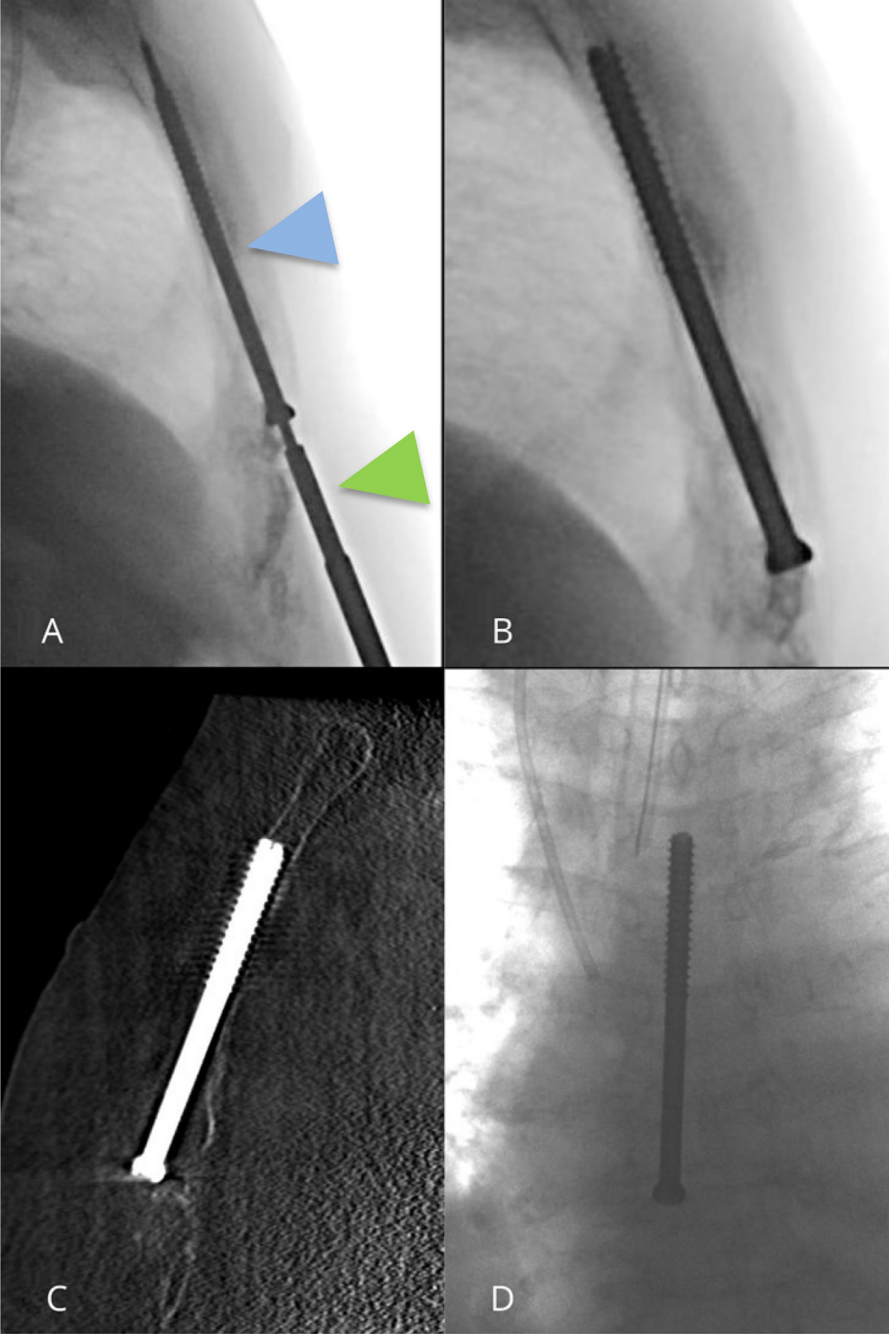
Lateral fluoroscopic control showing the partially threaded titanium Asnis III screw (Blue Arrowhead), on the Kirschner wire and the screwdriver (Green arrowhead) (A) Satisfactory screw position on fluoroscopy (B and D) Sagittal CBCT reconstruction showing the correct positioning of the screw (C).
